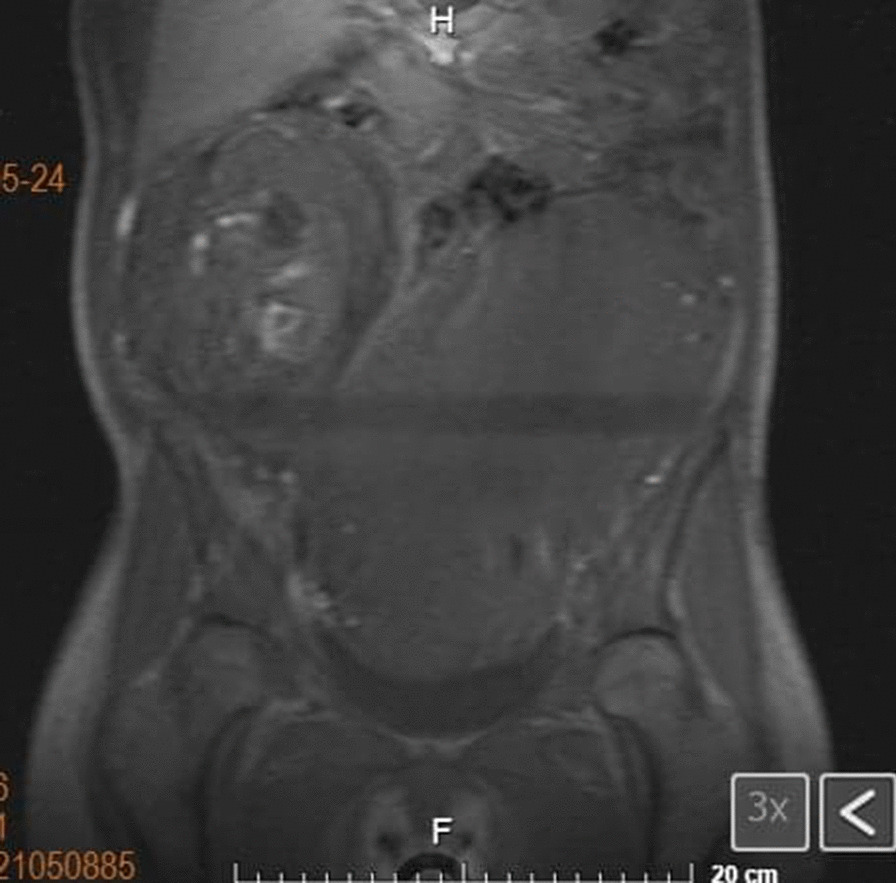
Abdominal-pelvic magnetic resonance imaging, fat-saturated T2-weighted sequence. Findings of the adnexal mass. Coronal view shows a heterogeneous left adnexal lesion, with regular lobulated contours, predominantly isointense to soft tissue, with approximate dimensions of 22.1 × 13.6 × 16.3 cm in its largest diameters
